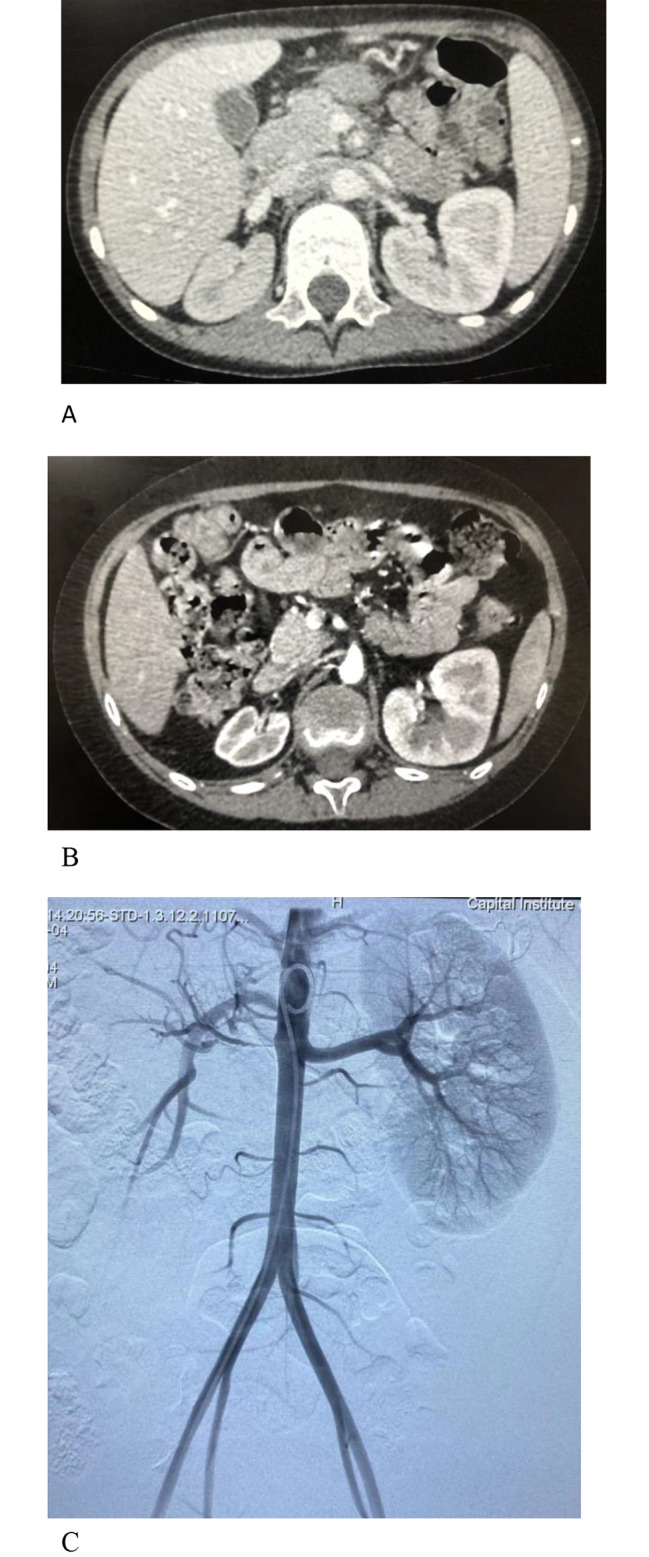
Patient 1, an 8-year-old girl, axial contrast-enhanced CT imaging. A showeds the narrowing of the right renal artery (arrow). Note the accompanying small renal size and deficient enhancement in the renal parenchyma. B 3 Three years later, a follow-up CT image demonstrated that the right kidney became further smaller had decreased in size. C This is an intraoperative imaging of the child, and the right renal artery was almost occluded so that there was very little blood supply to the right kidney, and the interventional guide wire was unable to pass through the stenosis
